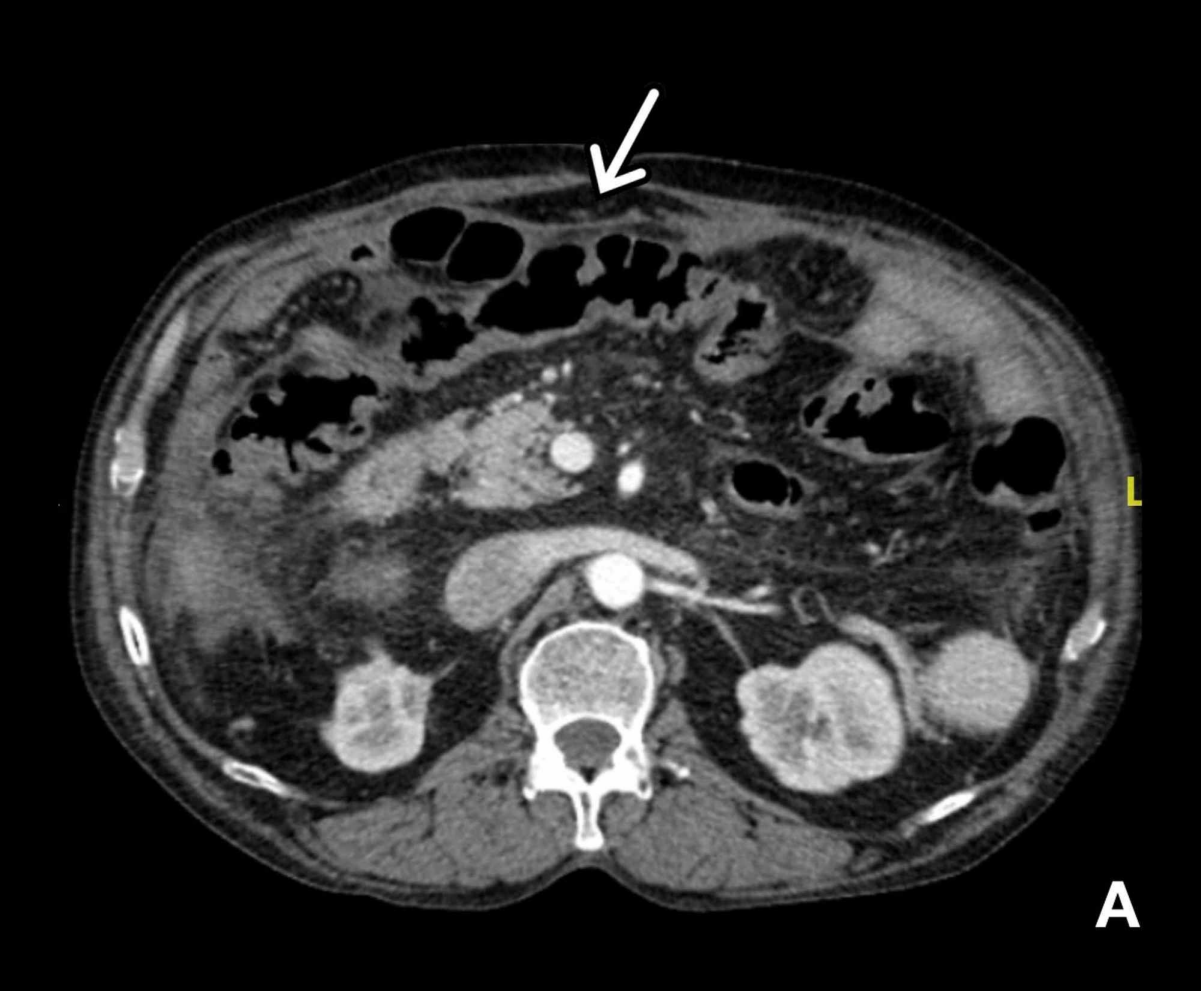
Axial view of CT of the abdomen, with arrow pointing to fibrous tissue encasing large bowel
